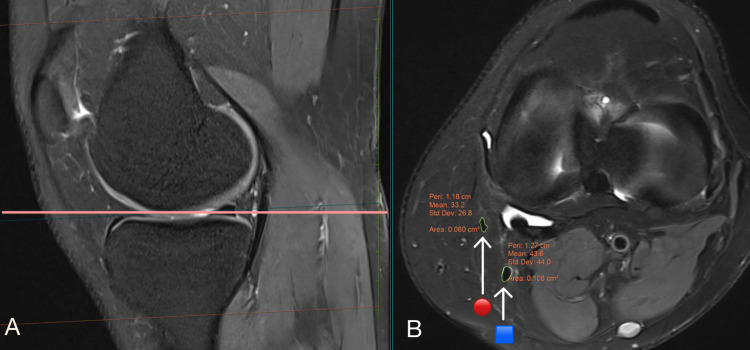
MRI scans showing (A) a sagittal view of the right knee joint; (B) an axial view of the right knee joint at the joint line, displaying the cross-sectional area of the semitendinosus and gracilis 🟦 denotes semitendinosus tendon; 🔴 denotes gracilis tendon
